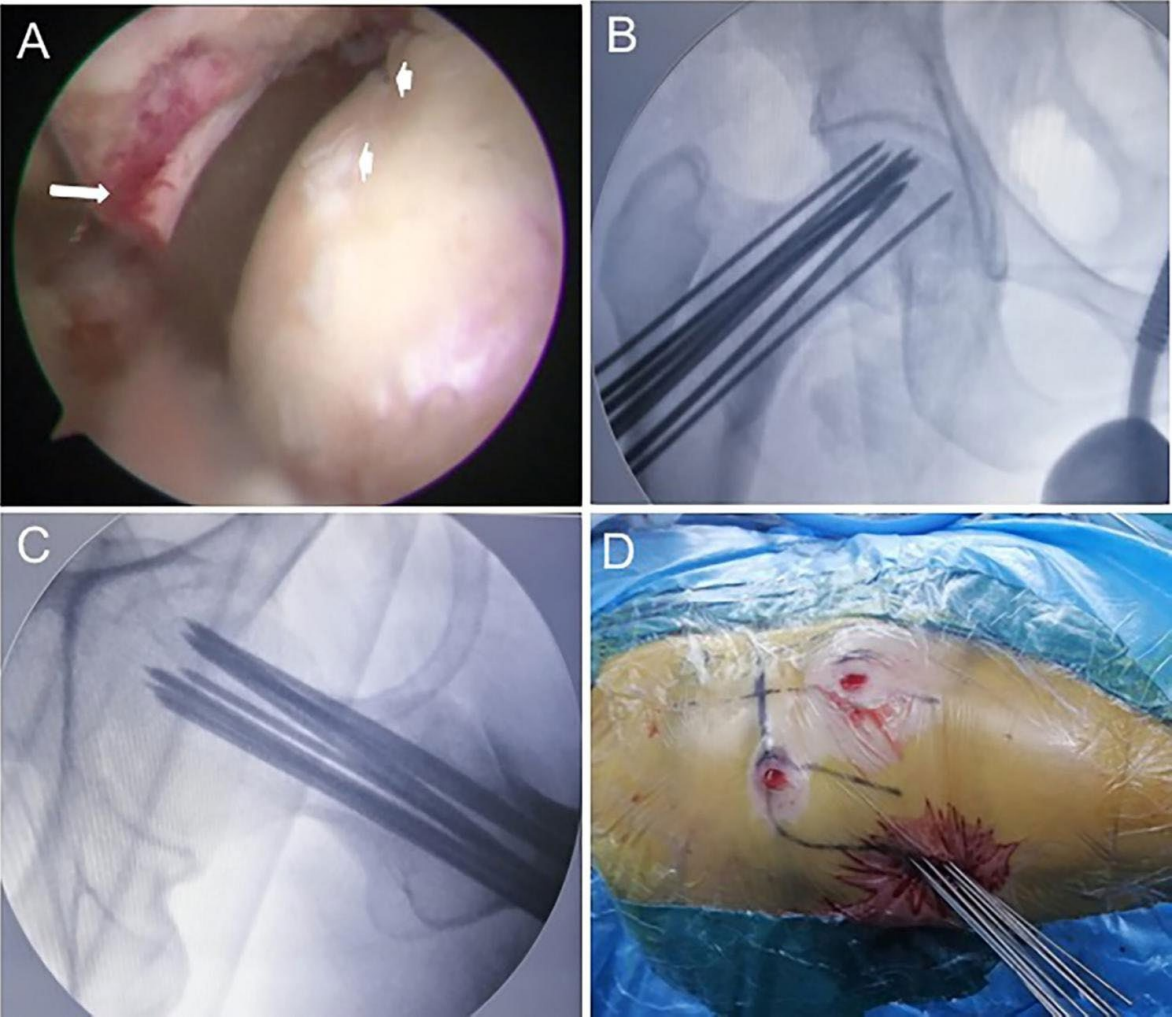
A Intraoperative imaging showed the congested synovium (white arrow) and cartilage fissure of femoral head (white arrows). B, C Intraoperative radiographic imaging showed pattern of multiple drilling decompression at proximal femur. D Intraoperative imaging showed percutaneous multiple drilling decompression of proximal femur
